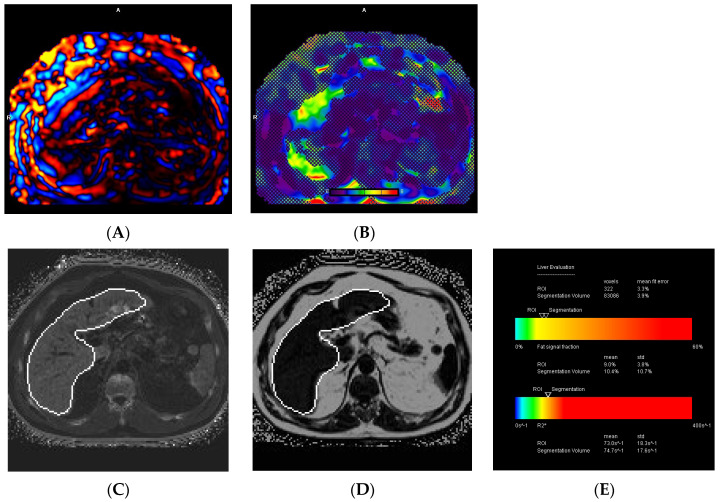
A 63-year-old male with HCV. Wave (A) and elastogram (B) MRE images show a heterogeneous fibrosis distribution equating to stage 2 to 3 fibrosis with a stiffness of 3.8 kPa. MRI-PDFF map (C), R2* map (D), and the liver evaluation table (E) demonstrate mild steatosis (10.4%) and borderline iron overload (R2* of 74.7 s^−1^ and a LIC of 2.4 mg/g) based on segmentation.
